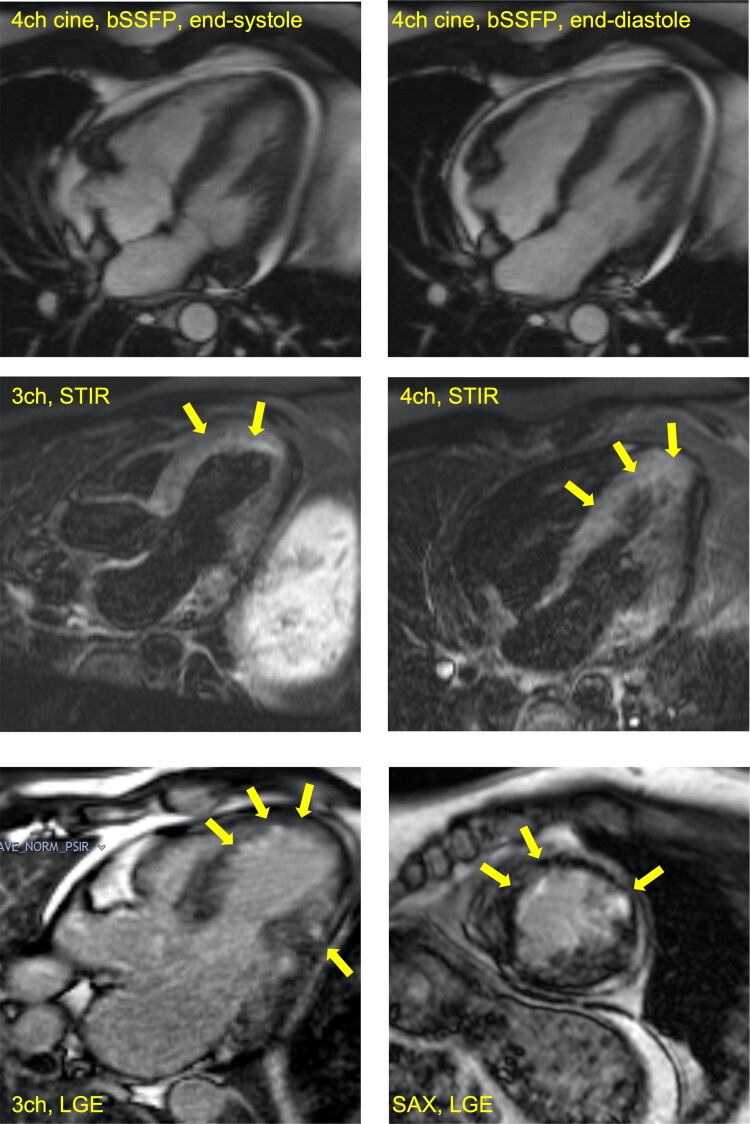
Key cardiovascular magnetic resonance images demonstrating myocardial oedema and subendocardial LGE without coronary distribution footnote. 3ch, three chamber; 4ch, four chamber; bSSFP, balanced steady state free precession; LGE, late gadolinium enhancement; SAX, short axis; STIR, short inversion time inversion recovery; Panels A and B. Four-chamber long-axis view frames at end-diastole (*A*) and end-systole (*B*) demonstrating reduced left ventricular function. Panel C. T2w-STIR-3 chamber showing enhancement in the anterior and anteroseptal segments. Panels C to D. EGE (early gadolinium enhancement) and LGE (late gadolinium enhancement) images demonstrating subendocardial contrast uptake in a non-ischaemic distribution.
